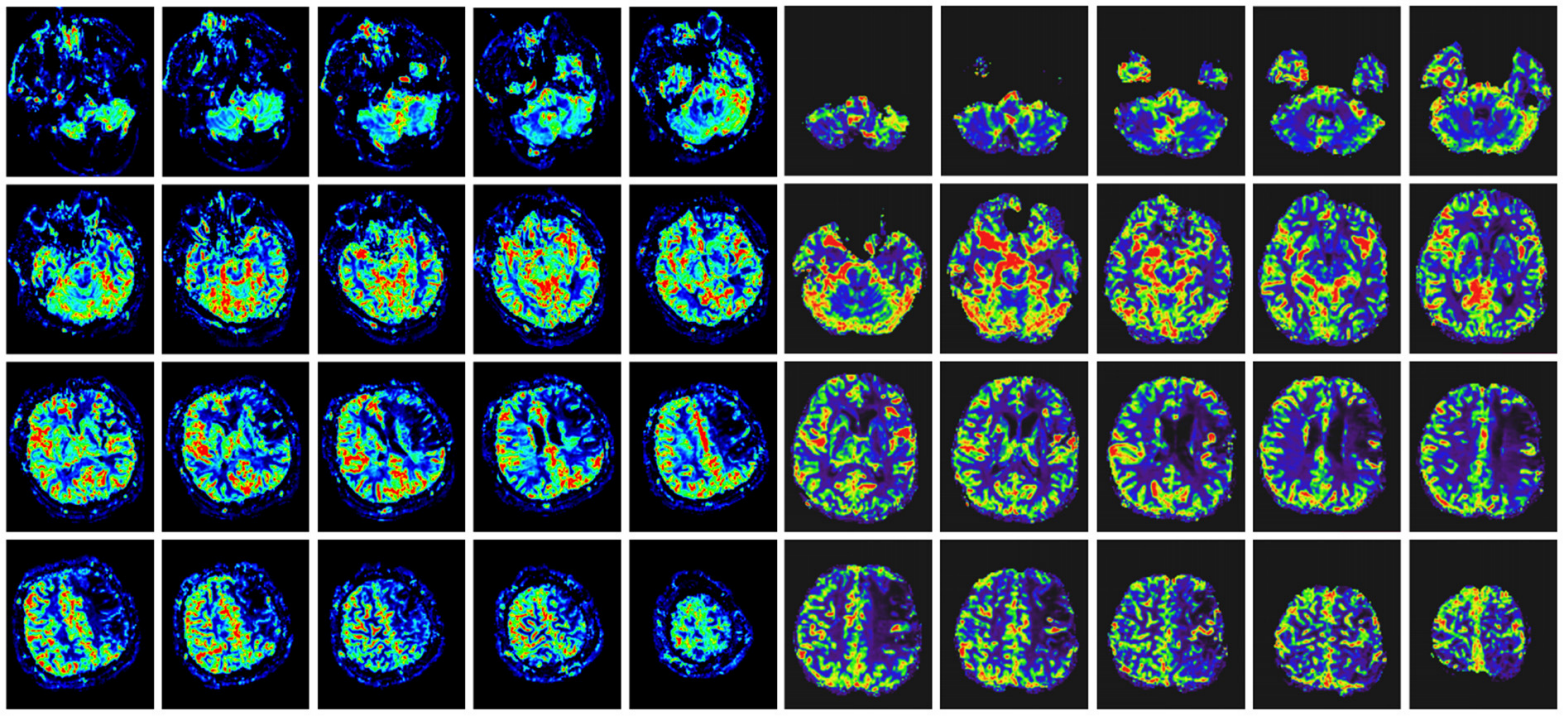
Comparison of head MRI perfusion before surgery (left) and after surgery (right) in the same patient with Figure 2. The preoperative ischemic region had improved perfusion in the same region after indirect bypass surgery.
